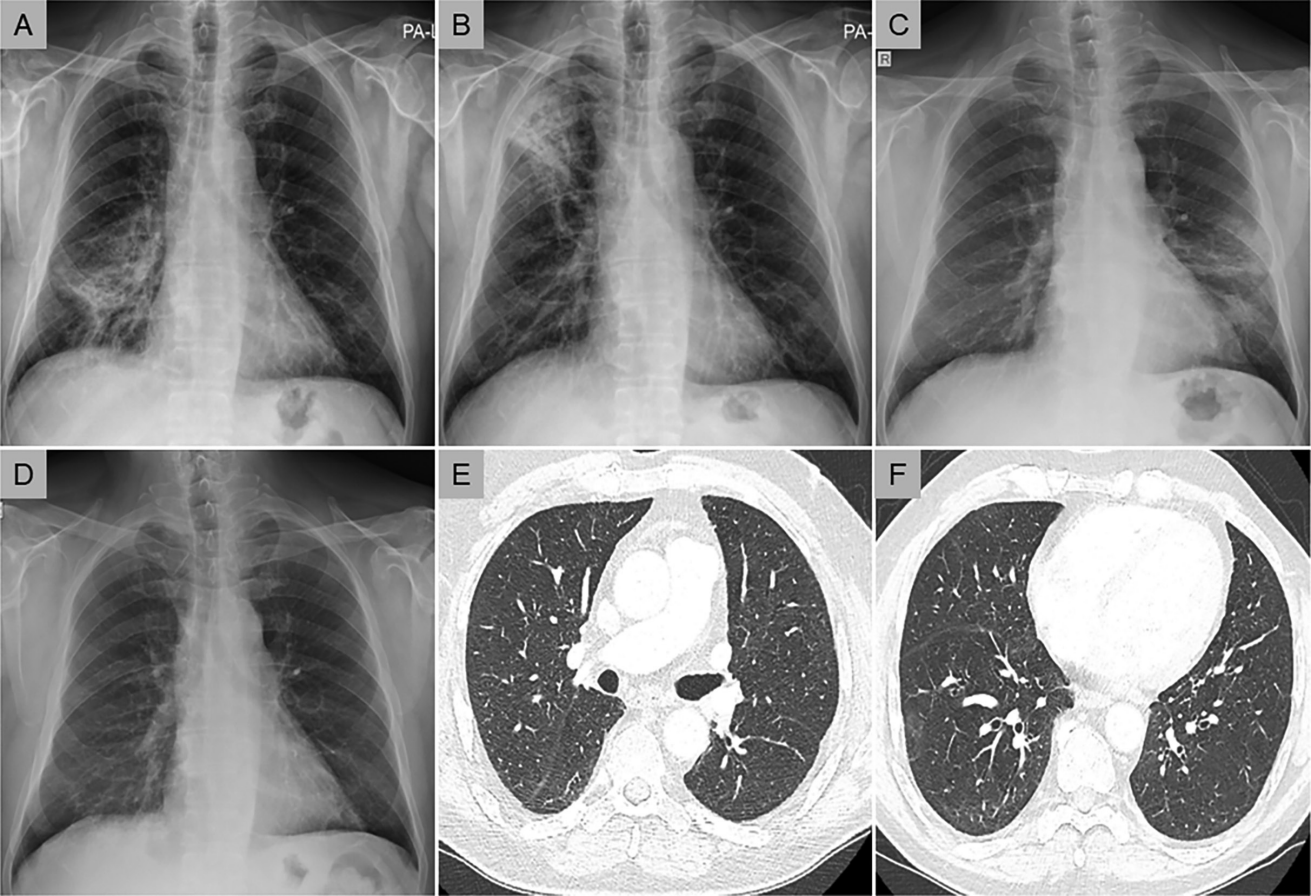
Radiological evolution and treatment response during disease course. (A–C) Serial chest radiographs during outpatient follow‐up showing recurrent migratory patchy infiltrates following attempts at steroid discontinuation. (D–F) Subsequent imaging after introduction of hydroxychloroquine demonstrating sustained resolution of opacities and maintenance of remission.
